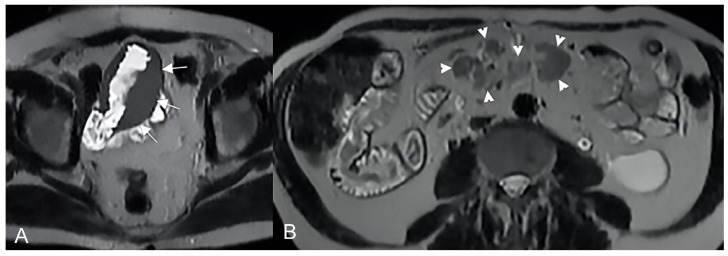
Example of Lymphoma in a 71-year-old male patient (the same patient in Figure 1C). (A) Axial T2-weighted image shows a marked asymmetric thickening of an ileal loop in the pelvis (arrows). (B) An axial T2-weighted image on a different plane shows multiple enlarged mesenteric lymph nodes (arrowheads).
